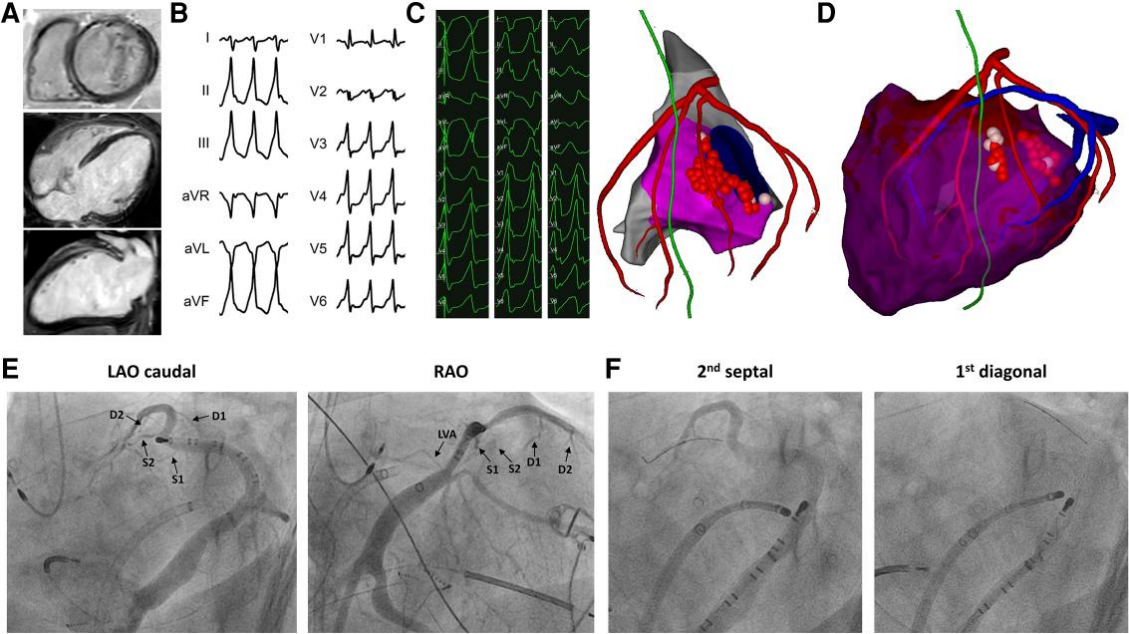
(*A*) Cardiac magnetic resonance images showing deep intramural late gadolinium enhancement around the mitral annulus. (*B*) A 12-lead echocardiography showing ventricular tachycardia with an inferior axis, rS pattern in V1-2, and sudden QRS complex transition in V3. A slow upstroke of the QRS complex suggests epicardial origin. Collectively, this indicates left ventricular summit tachycardia. (*C*) Anatomical and bipolar endocardial maps fused with segmented cardiac computed tomography images showing ablation sites with the successful elimination of three ventricular tachycardias. (*D*) Anatomical and bipolar epicardial maps fused with segmented cardiac computed tomography images showing a limited ablation set due to the inter-position of the phrenic nerve and coronary artery. (*E*) Left anterior oblique caudal and right anterior oblique images of coronary sinus angiography. LVA, left ventricular annular branch; S1, first septal branch; S2, second septal branch; D1, first diagonal branch; D2, second diagonal branch. (*F*) The left fluoroscopy image shows a selective distal cannulation of the second septal branch with a unipolar pacing wire and over-the-wire balloon. Here, the pace-match was 97% similar to the clinical tachycardia with a precocity of −52 ms to the onset of the QRS complex. The right fluoroscopy image shows a selective cannulation of the first diagonal branch with the unipolar pacing wire and over-the-wire balloon, showing similar excellent pace-match and precocity parameters.
